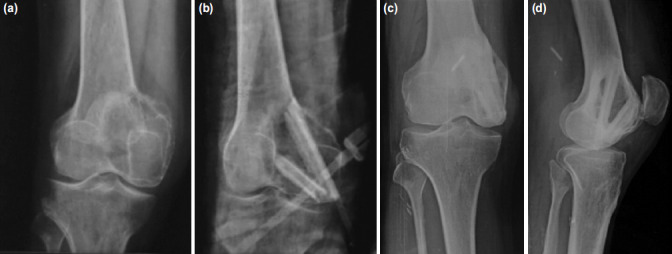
(a) Giant cell tumour of the medial femoral condyle in a 38-year-old. (b) Immediate post-operation radiograph shows cavity supported with two struts. (c,d) Good hypertrophy and incorporation of the graft at two years post-operation.
